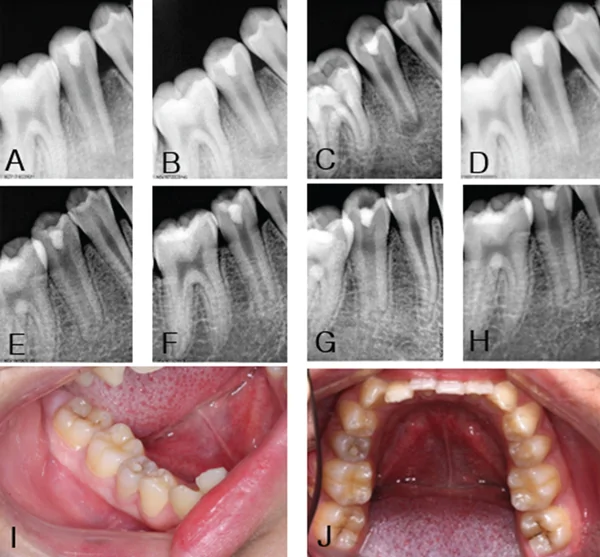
(A) First available radiograph obtained immediately after initial treatment; (B) 1‐month postoperative radiograph; (C) 3‐month postoperative radiograph; (D) 7‐month postoperative radiograph; (E) 10‐month postoperative radiograph; (F) 16‐month postoperative radiograph; (G) 19‐month postoperative radiograph; (H) 31‐month postoperative radiograph; and (I,J) intraoral photograph at the 31‐month follow‐up.
